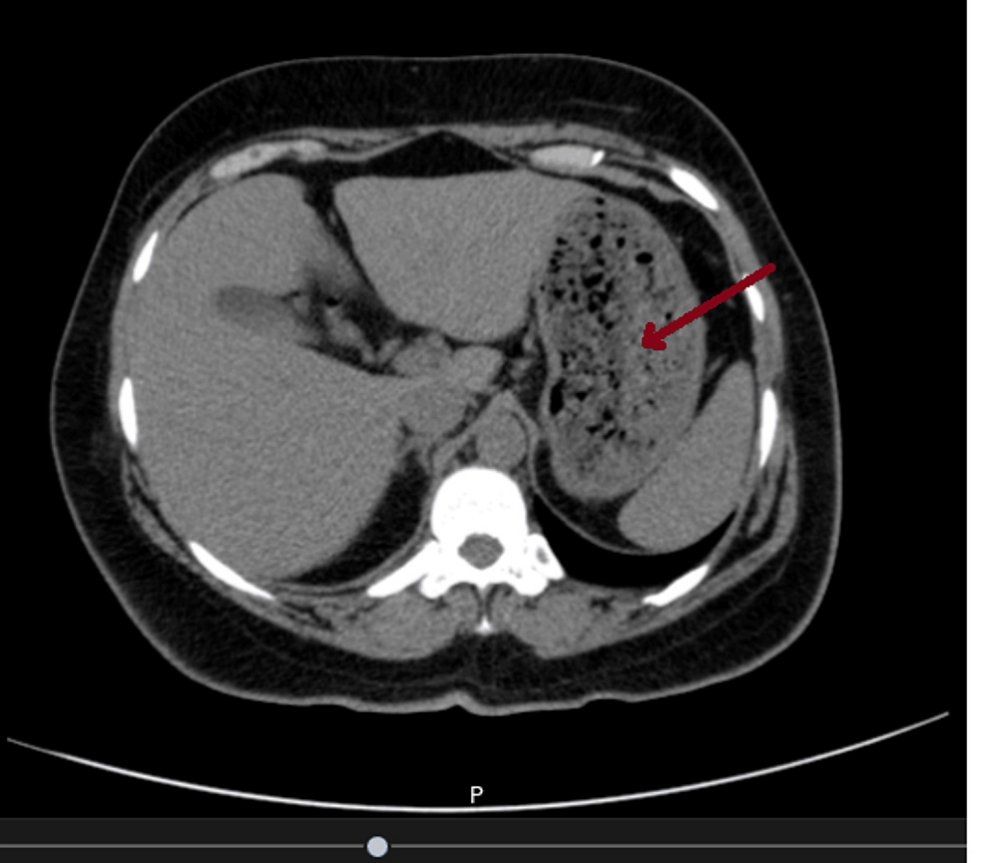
CT scan image showing the patient's stomach filled with material resembling a bezoar.
